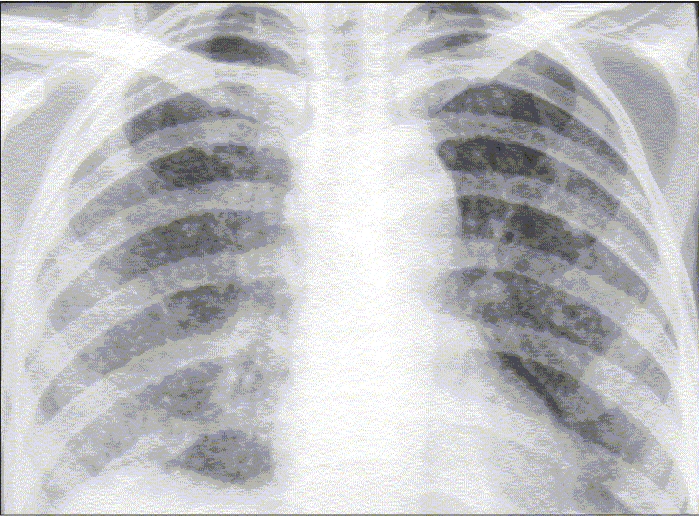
Chest radiograph frontal view showing miliary opacities over bilateral lung fields.
